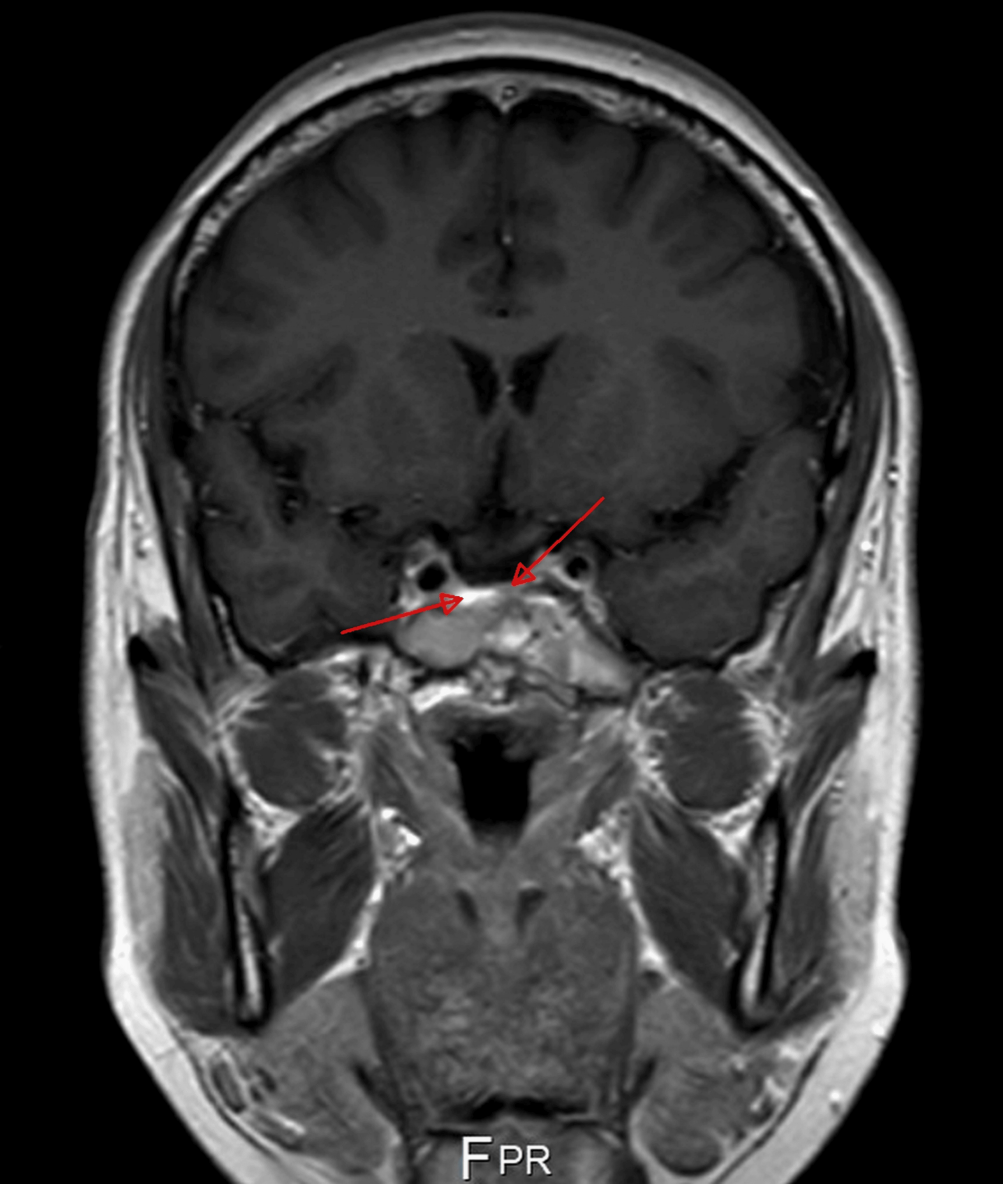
MRI with contrast showing resolution of the lesion six months after the surgery
